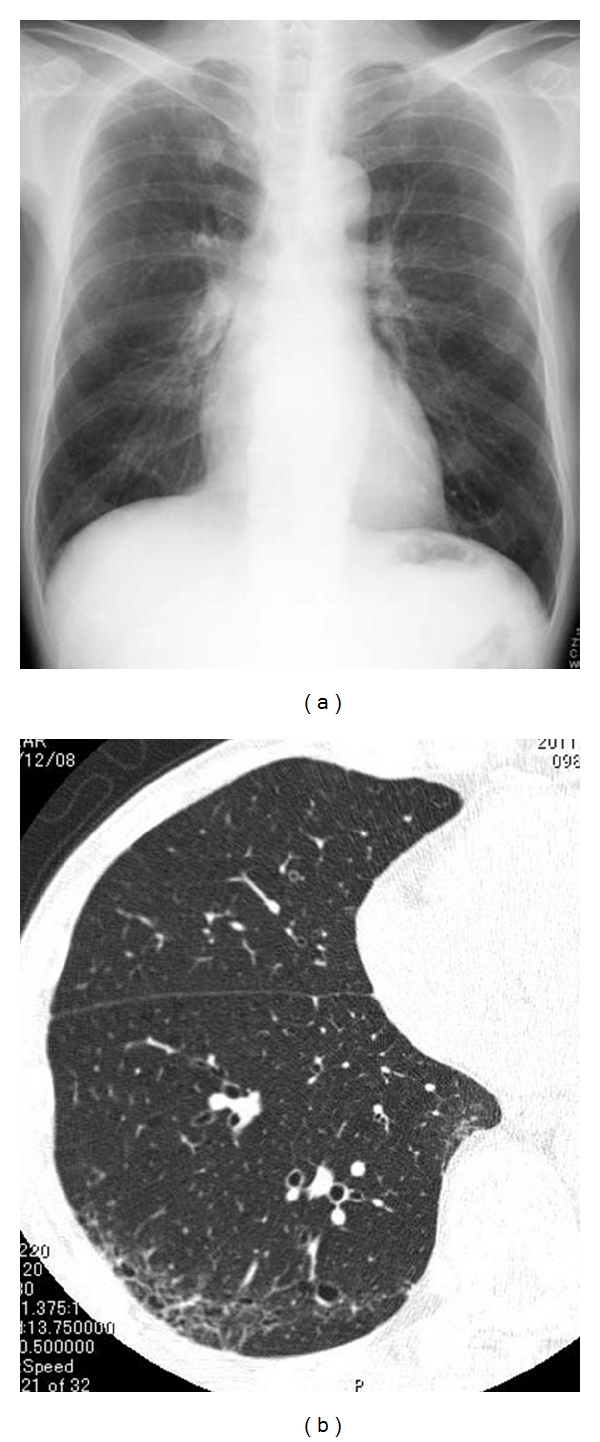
Airway-centered fibrosis with cysts. (a) Chest X-ray shows increased volume of bilateral lungs. (b) High-resolution computed tomography (HRCT) of lower lung shows ground glass opacity and multiple cysts in subpleural region, but these abnormal opacities are predominant in centrilobular region (centrilobular accentuation).
